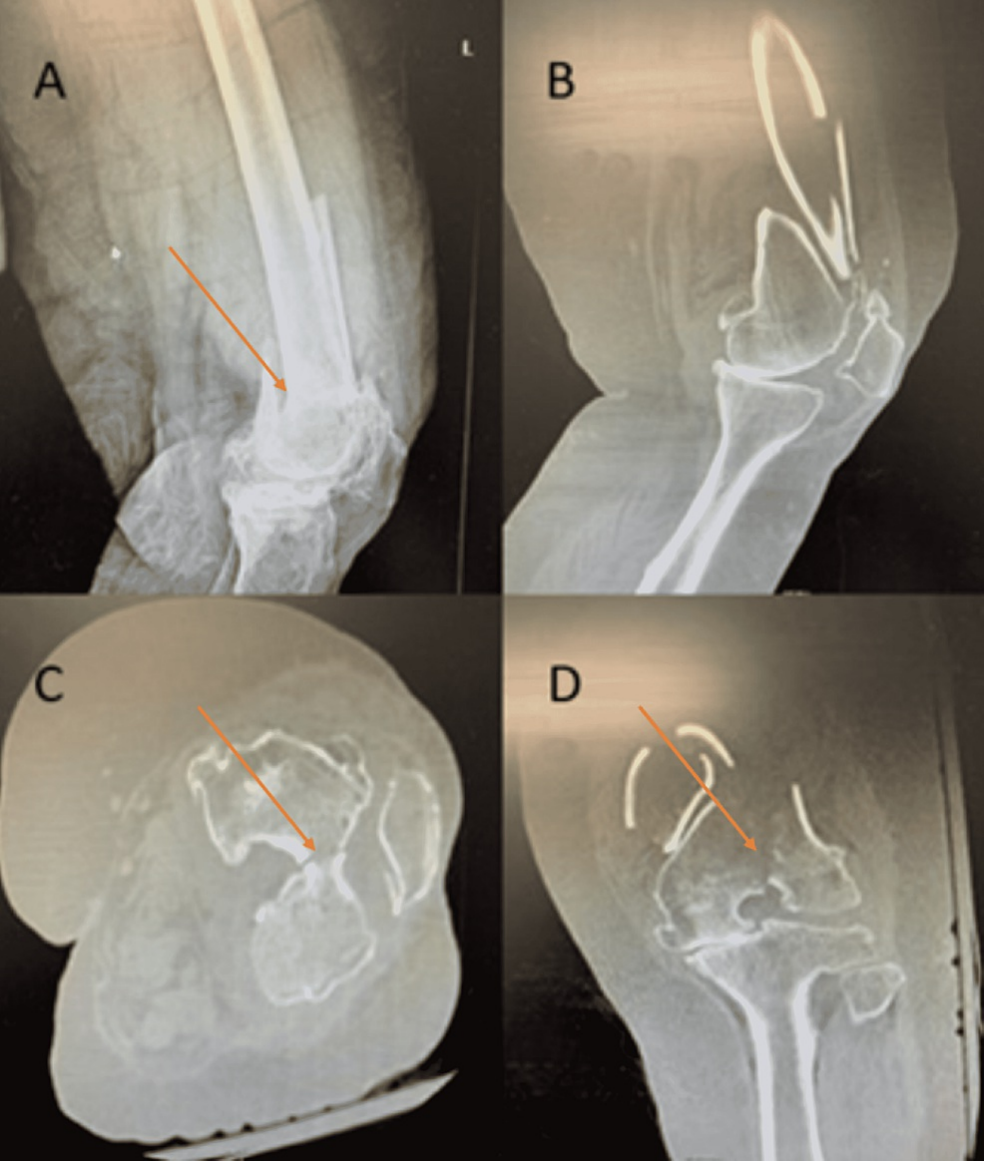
Preoperative imaging revealing OTA class C3 fracture of the left distal femur. A: Lateral X-ray of the left knee. B: Lateral CT of the left knee. C: Axial CT of the left knee. D: Coronal CT of the left knee. Arrows dictate intercondylar extension of the fracture with intact lateral wedge consistent with OTA C2.1 fracture classification. CT: computed tomography
